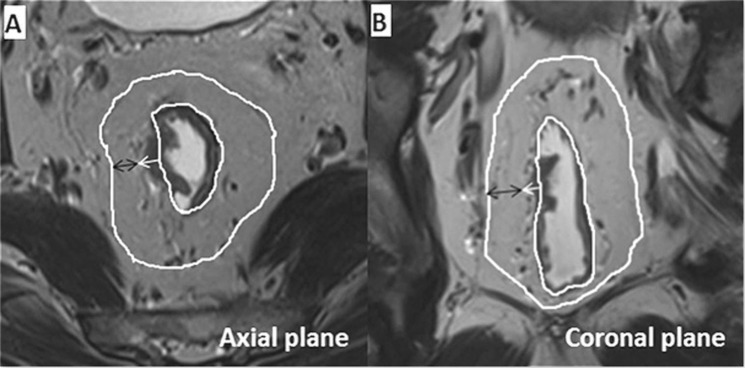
T2-weighted axial (A) and coronal (B) magnetic resonance images. Magnetic resonance imaging-detected the depth of mesorectal extension (MR-DME) ≤ 4 mm and negative MRF. The black double-headed arrow indicates the minimum distance from the tumor to the mesorectal fascia, and the white single-headed arrow indicates the MR-DME. The white line indicates the mesorectal fascia and the muscularis propria.
